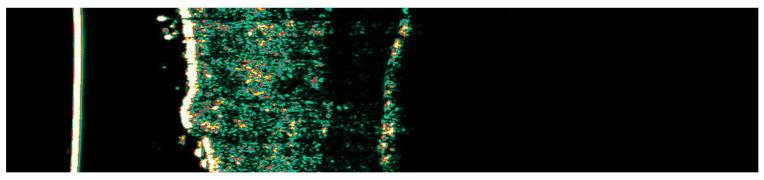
Ultrasonographic image of sebaceous hyperplasia.
